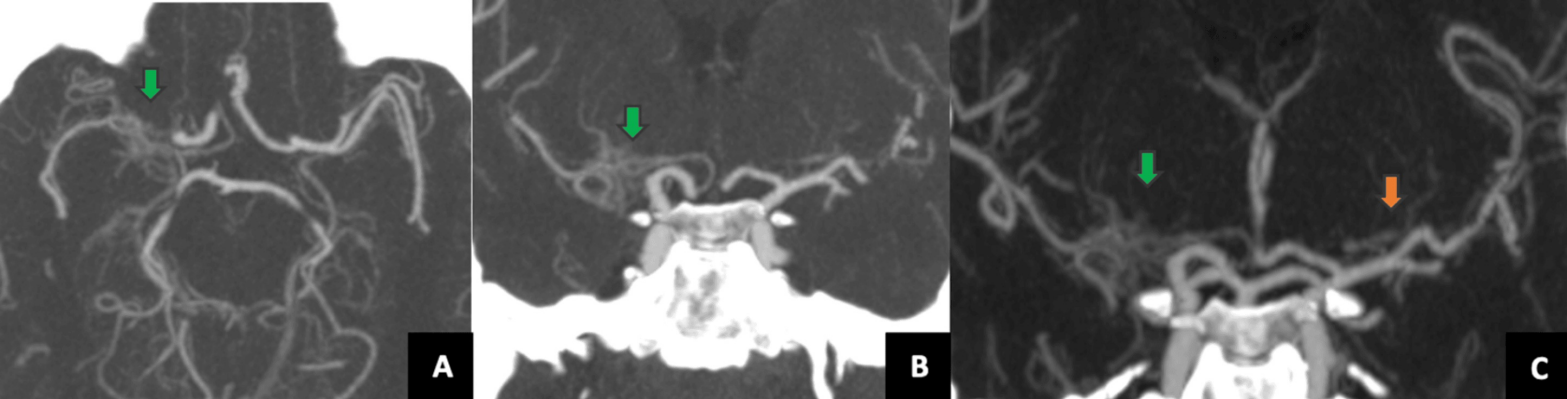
CT angiography in axial (A) and coronal planes (B and C) and maximum intensity projection (MIP) The CT angiography revealed an abnormal plexiform network (A and B) in the right M1 segment (green arrow) and early division of the left middle cerebral artery with trifurcation (C, orange arrow).
